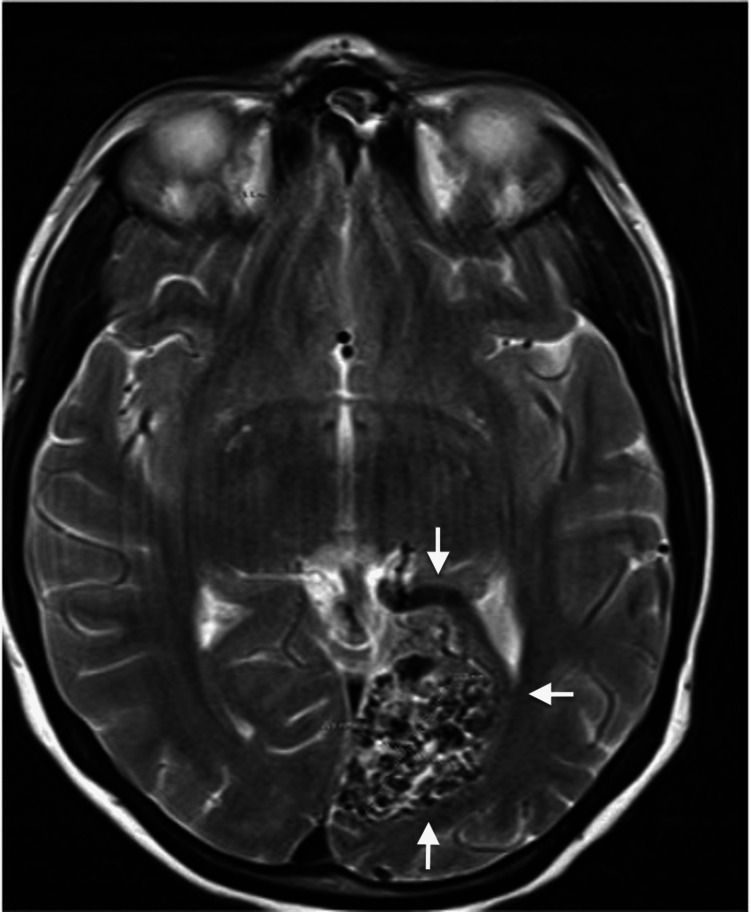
Axial T2-weighted MRI of the brain demonstrating an arteriovenous malformation (AVM) in the medial left occipital lobe involving the calcarine cortex (arrows), measuring approximately 2.9 cm. No evidence of intracranial hemorrhage is present. Minimal perilesional gliosis is noted adjacent to the AVM.
